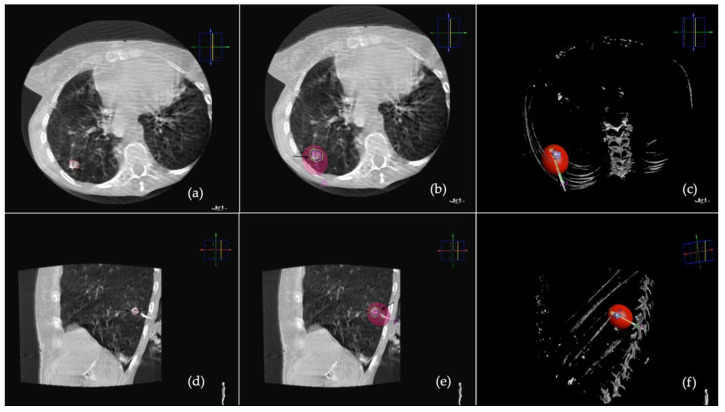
VPS was applied to CBCT images. (a) On axial and (d) sagittal CBCT scans the tumour has been segmented. (b) On axial and (e) sagittal CBCT scan, MWA is placed over the virtual one, the predicted ablation volume is seen as a purple ellipsoid and the green line (black arrow) shows that the predicted volume entirely encompasses the segmented lesion. (c) Axial and (f) sagittal 3D reconstruction of the lesion and predicted ablation volume.
